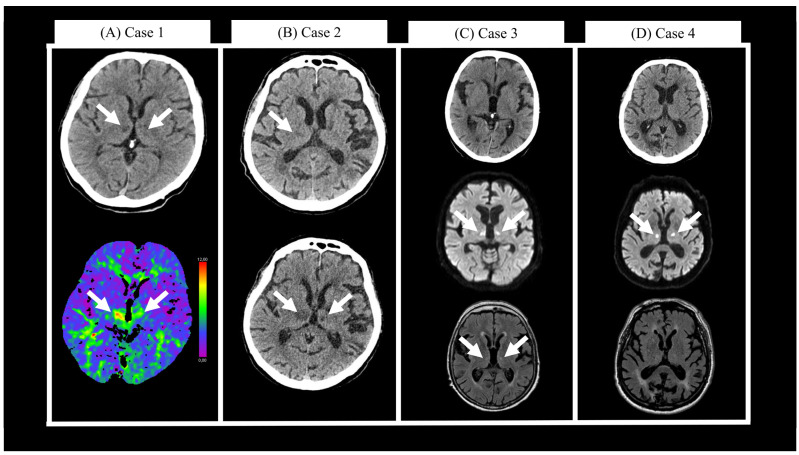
(A) Case 1: head computer tomography (CT) (upper) and CT perfusion (lower) scans two days after initial presentation. (B) Case 2: head CT scans at initial presentation (upper) and one day later (lower). (C) Case 3: head CT scan at initial presentation (upper), magnetic resonance imaging (MRI) diffusion weighted imaging (middle), and fluid attenuated inversion recovery (lower) at initial presentation. (D) Case 4: head CT (upper), MRI diffusion weighted imaging (middle), and fluid attenuated inversion recovery (lower) at initial presentation. White arrows show changes in the thalami.
